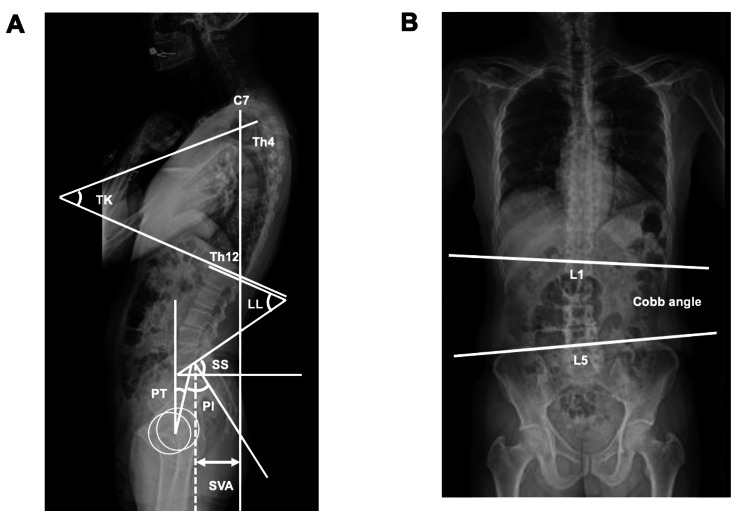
Measurements of radiological parameters Lateral (A) and anteroposterior (B) standing radiographs SVA: sagittal vertical axis; TK: thoracic kyphosis angle; LL: lumbar lordosis; SS: sacral slope; PT: pelvic tilt; PI: pelvic incidence; Cobb angle: between the inferior endplates of L1 and L5
